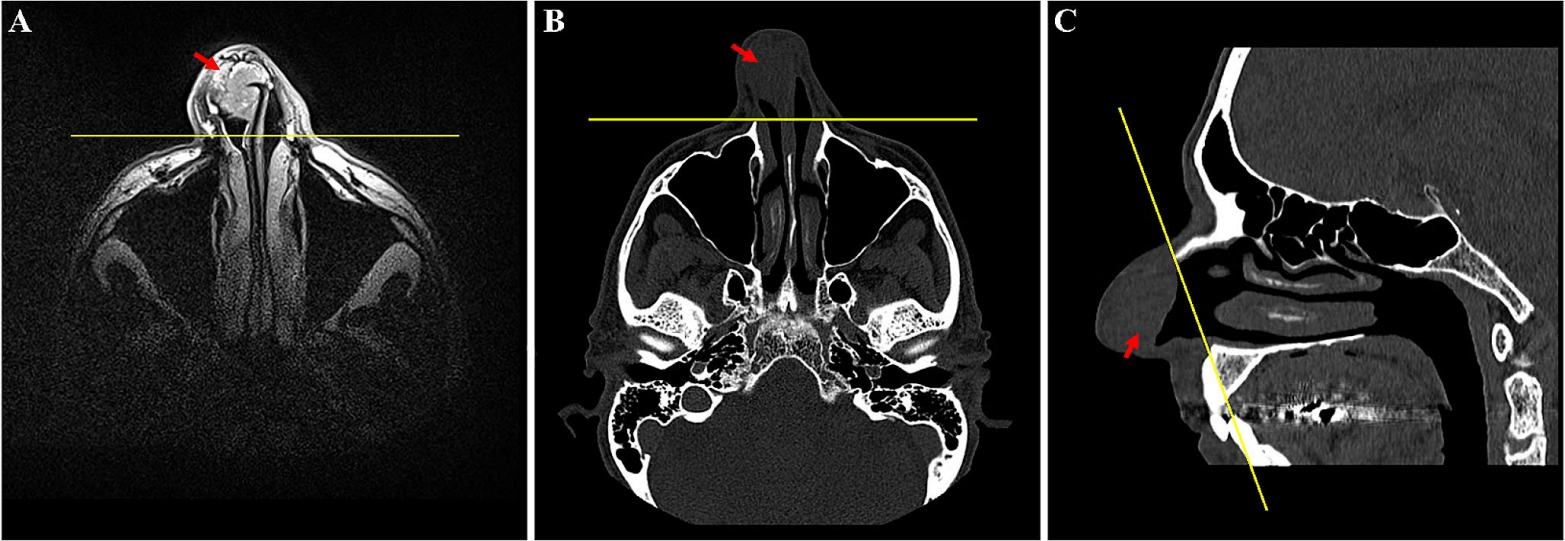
Pretreatment imaging of a patient diagnosed with a right NVSCC, limited to the NV, anterior to a line passing through the piriform aperture, staged as T1 according to Wang and T2b according to Bussu et al. [21]. Axial MRI T2-weighted sequence (A) shows septal cartilage infiltration of the tumor. CT-scan images (axial, B and sagittal, C) allow clear identification of the bony piriform aperture
